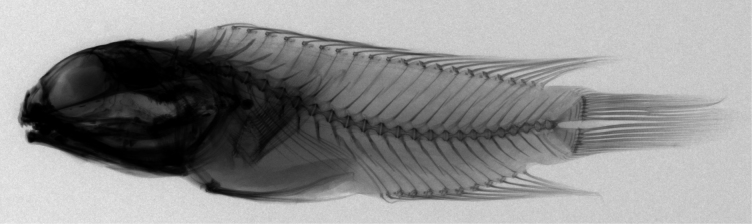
X-radiograph of *Opistognathuscryos* sp. nov., holotype, NMMB-P 36179, 65.1 mm SL.
